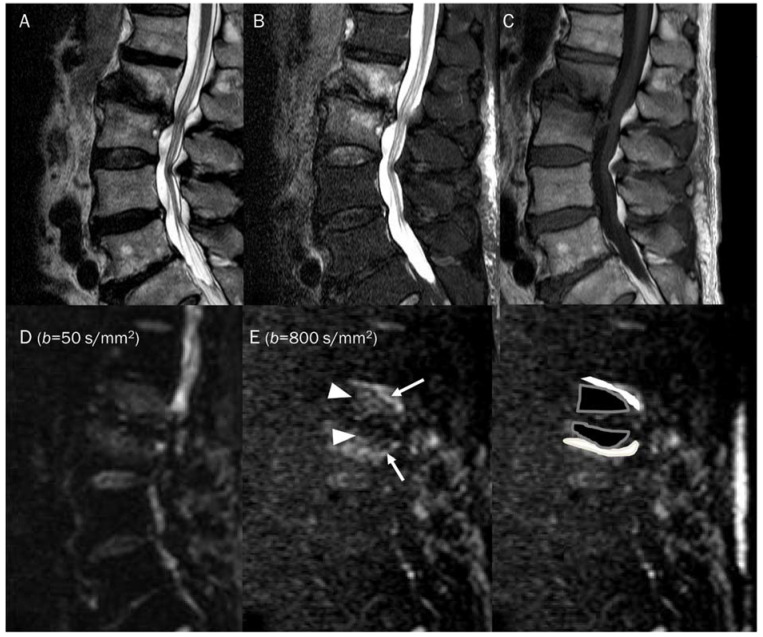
A 81-year-old male with type 1 Modic change in the endplates of L2-3. Conventional sagittal MRIs (A: T2-weighted, B: T2-weighted fat-suppressed, C: T1-weighted) show an indistinct bone marrow edema in the adjoining endplates of L2-3. (D,E) DWI with *b*-values of 50 and 800 s/mm^2^ exhibit persistent outer high signal bands (arrows) with inner low signal areas (arrowheads), displaying a ‘claw sign’, indicative of type 1 Modic change. The inner low signal areas may be due to the T2 black-out effect caused by fatty marrow.
